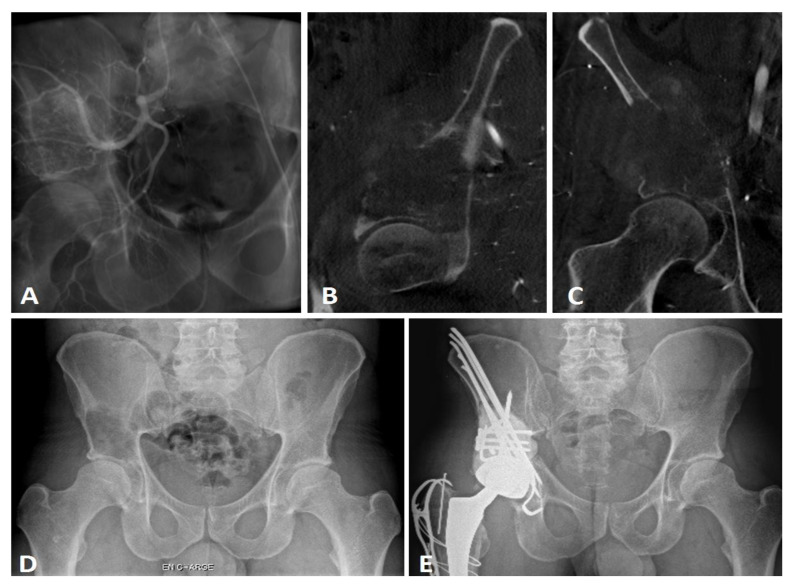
Imaging views of a GIST metastatic iliac lesion Harrington class III. Scout view (A) during the preoperative embolization, sagittal view (B) of the periacetabular lesion, frontal view (C). X-ray AP view of the pelvis before surgery (D), and postoperative X-rays at 18-month follow-up (E).
